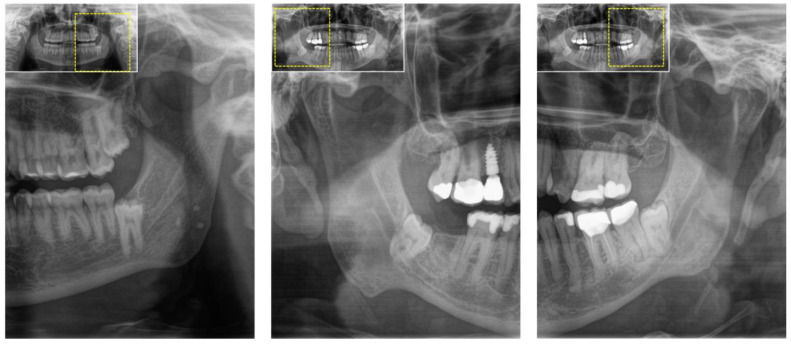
Two-dimensional orthopantomography (OPG) was initially performed in patients with a clinical indication for third molar surgery. This radiographic assessment reveals several radiographic risk signs, including the overlap of the third molar and mandibular canal, as well as incomplete of the osseous boundaries of mandibular canal. In such high-risk cases, three-dimensional imaging, such as cone-beam computed tomography (CBCT), is recommended to improve preoperative assessments and refine surgical planning.
